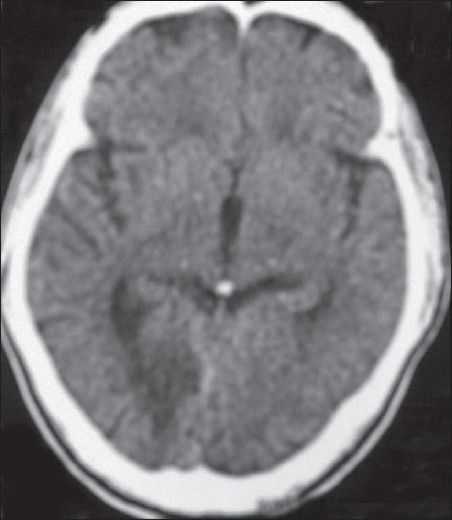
CT brain – right occipital infarct
